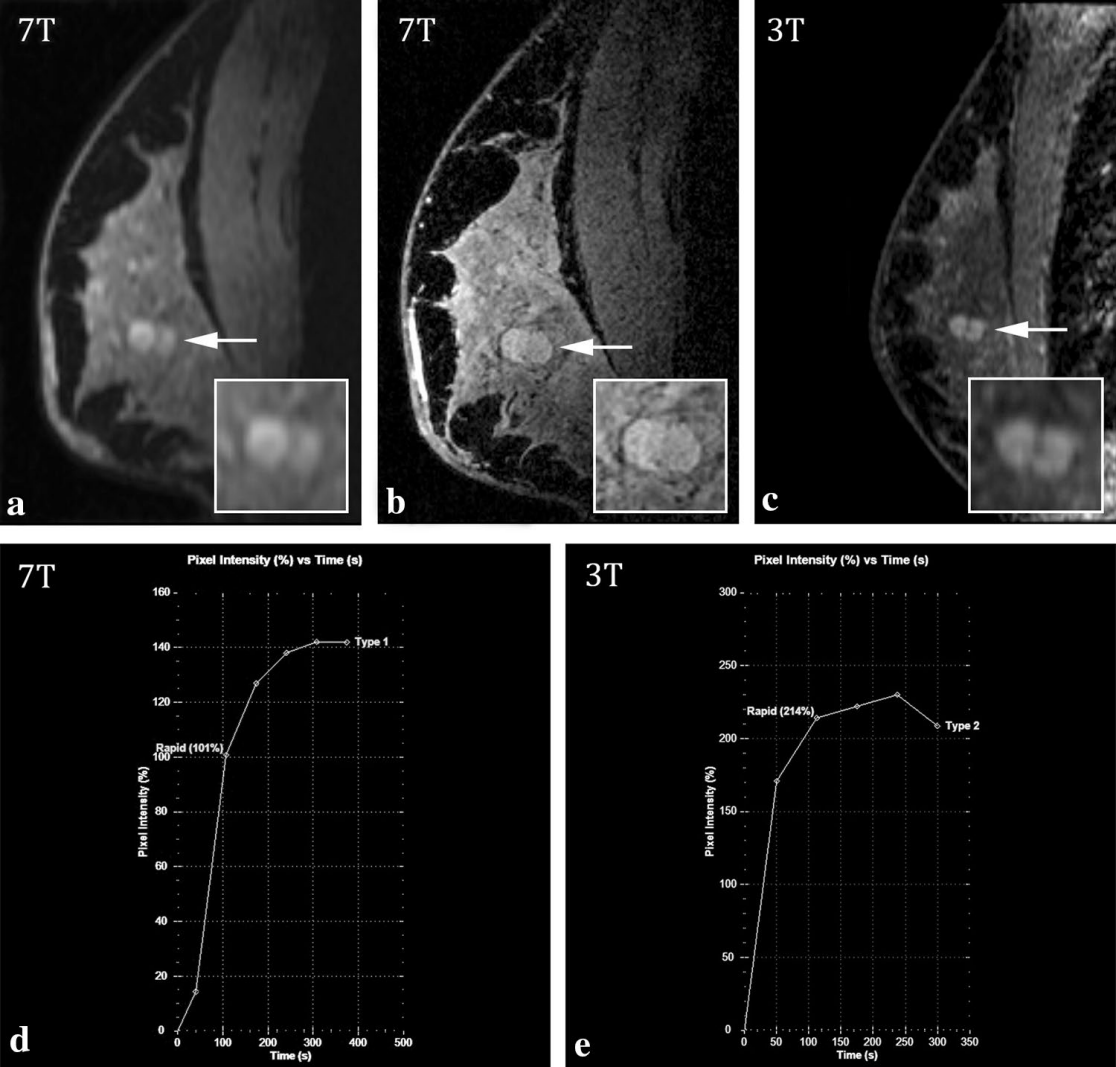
7T (a, b, d) and 3T (c, e) MRI images of a 65-year-old female patient. The depicted lesion in her right breast was diagnosed as fibrocystic changes after biopsy. Sagittal images of 2nd post contrast-injection series (a, c) show a lobular lesion (*arrow*) with irregular (R1 at 3T) or smooth margins (R1 at 7T and R2 at 3T and 7T). *Inset* shows zoomed-in image. Ultra-high resolution 7T image of the same slice (b). The kinetic curve assessment at 7T shows a rapid rise and persistent pattern in the delayed phase (d), and at 3T a rapid rise and plateau pattern (e). R1 classified the lesion as BI-RADS 3, and R2 as BI-RADS 3 (3T) and BI-RADS 2 (7T)
